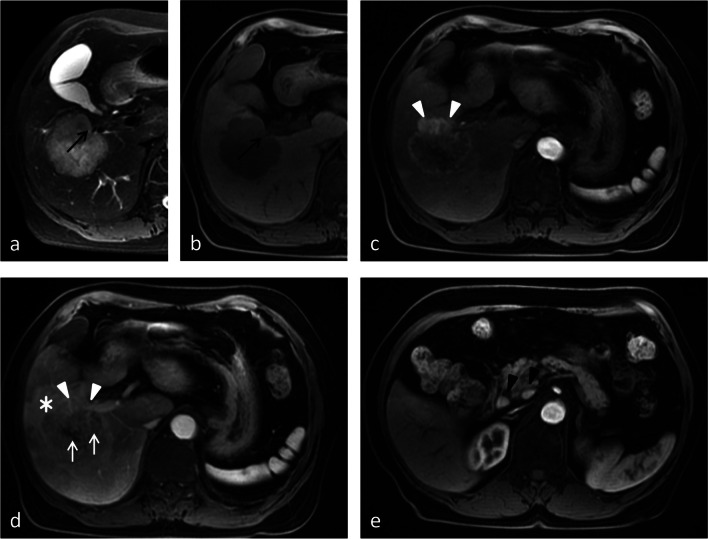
Images from a 70-year-old female with IMCC. a The T2-weighted image and (b) T1-weighted image show a 5-cm lesion in segment V-VI of the liver with hepatic capsular retraction (black arrow). c The arterial phase image shows partial hyperenhancement (white arrowhead). d The portal venous phase image shows continuous partial enhancement (white arrowhead), peripheral hepatic enhancement (star), and vessel penetrating the tumor (white arrow). e The portal venous phase image at a lower level shows the enlarged lymph nodes around the pancreatic head (black arrowhead). Thus, a score of 0 was assigned for this patient
